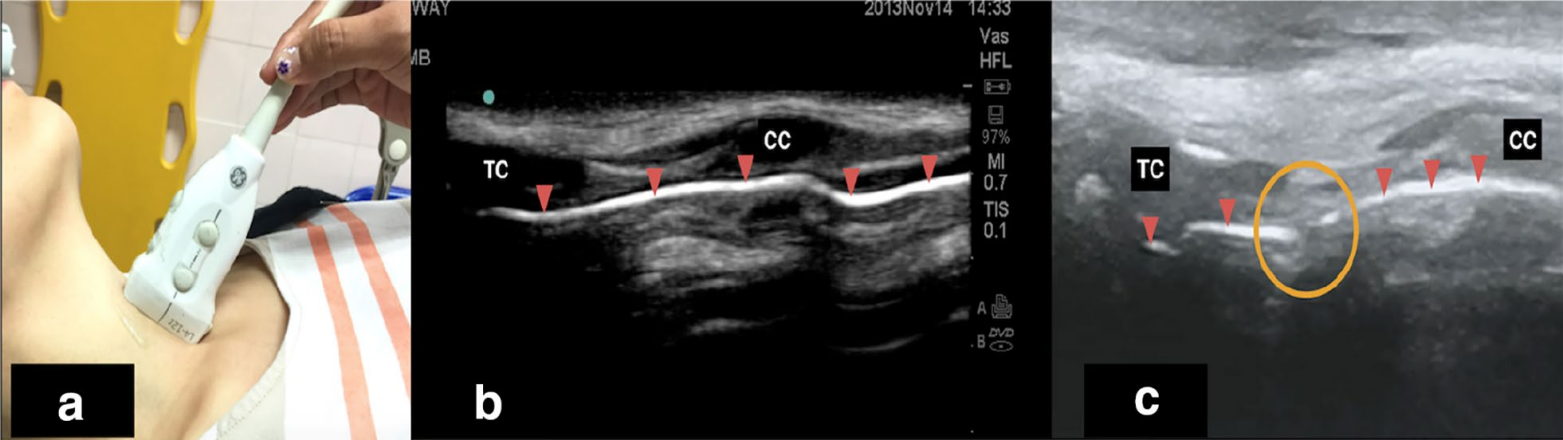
a Surface landmark of probe position (in longitudinal view) in relation to scanning the area for b and c. b Normal sonoanatomy of upper airway showing the relationship between thyroid cartilage, cricoid cartilage, air–mucosal interface and surrounding soft tissues. A continuous and undisrupted line of air–mucosal interface seen as hyperechoic line (arrowhead) c Airway ultrasound showed disruption air–mucosal interface without obvious detectable laryngeal fracture (circle). SM: sternocleidomastoid muscle; TC: thyroid cartilage; CC: cricoid cartilage
